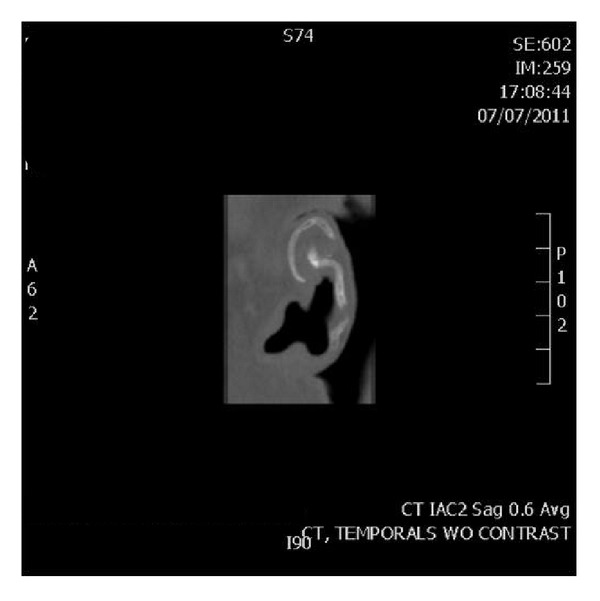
Computed tomography scan of left ear displays partial ossification of the external auricular cartilage.
